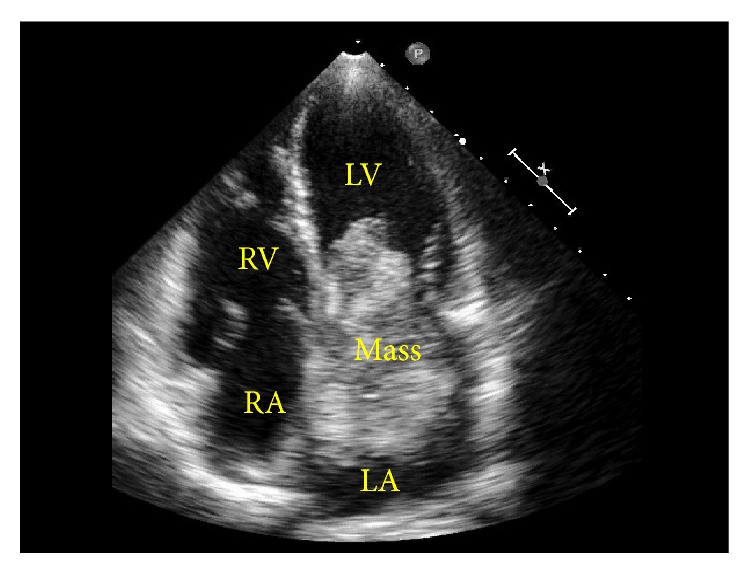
Echocardiography before surgical resection. Transesophageal four-chamber view showed a pedunculated mobile mass in the left atrium arising from the atrial septum. There was no thrombus in the left atrial appendage. LA: left atrium, LV: left ventricle, RA: right atrium, and RV: right ventricle.
